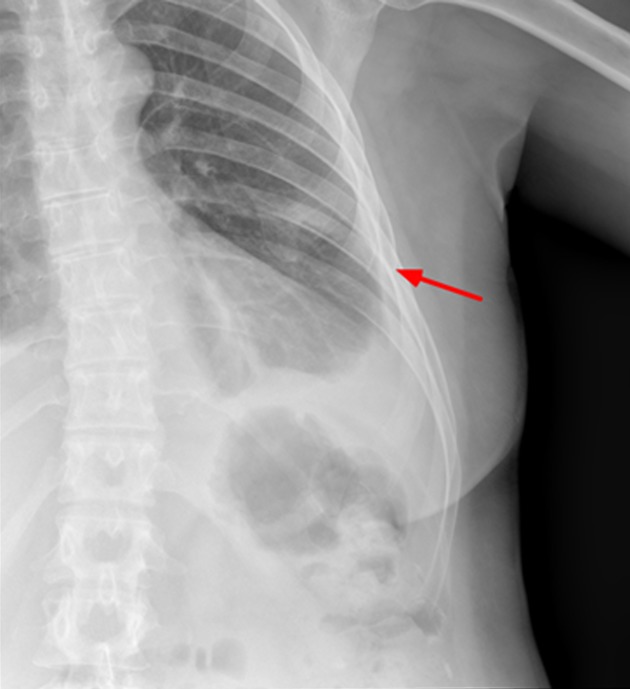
X-ray of left rib cage which may show partial disappearance of the 6^th^ left lateral and anterior rib arch.
